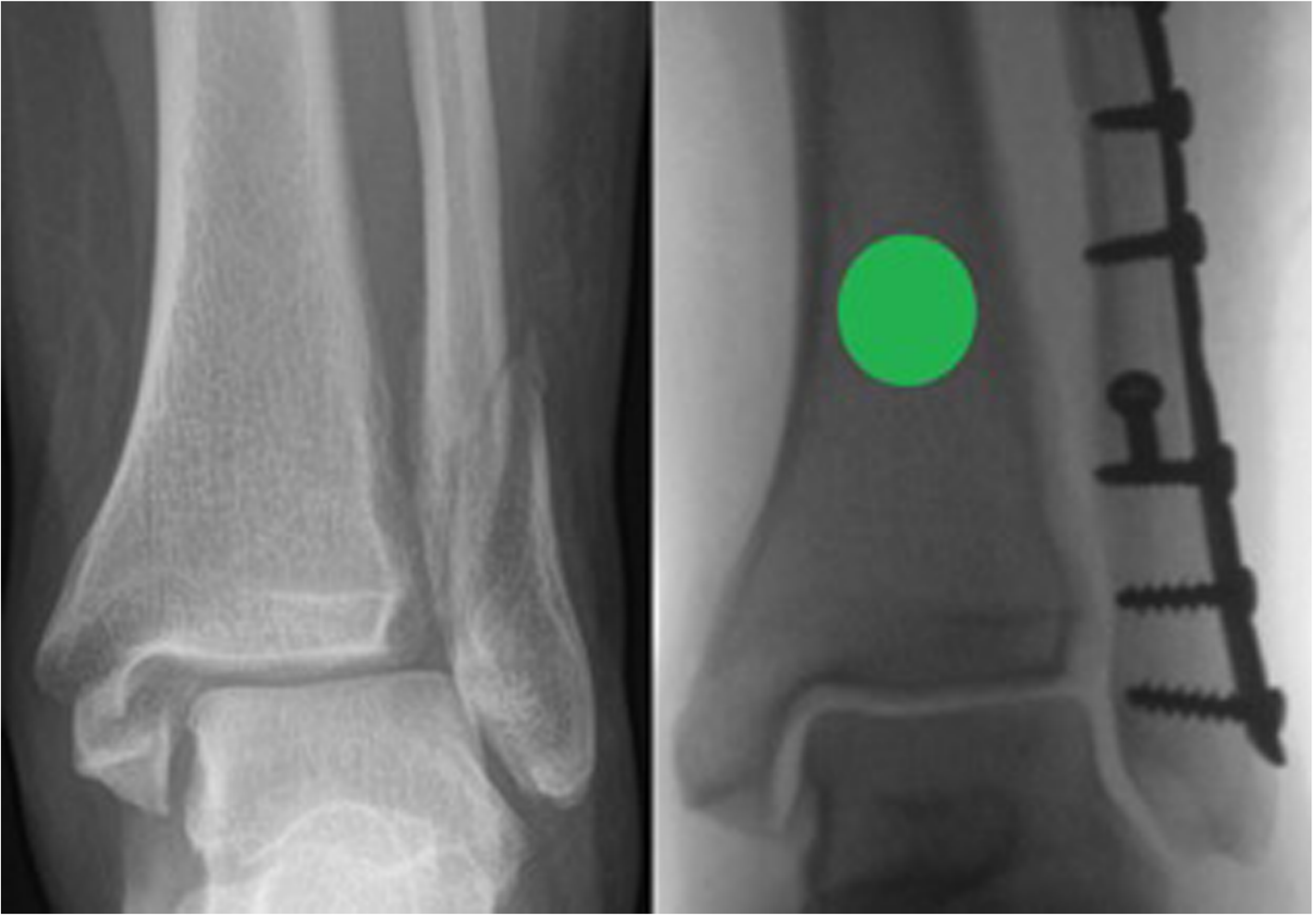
Anteroposterior radiograph of a supination-external rotation stage IV fracture at the time of injury and following fibular stabilisation, demonstrating an anatomically reduced medial malleolus. This patient would be eligible for intra-operative randomisation
